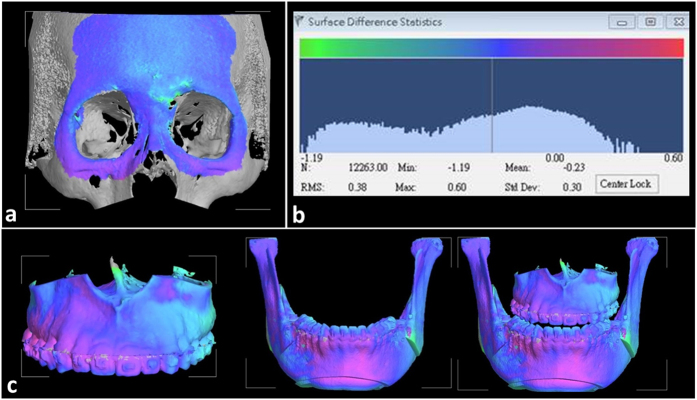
The discrepancy of superimposition of models was represented in the color-coded visualization and presented in terms of RMSD value (a). Initial registration of virtual planning and postoperative CBCT model (b). The RMSD distribution and statistics (c). The discrepancy of superimposition of virtual surgery and postsurgical images in maxilla, mandible and maxilla-mandibular complex segments. The blue color indicated the no deviation on both surfaces, other colors showed different degree of deviation.
